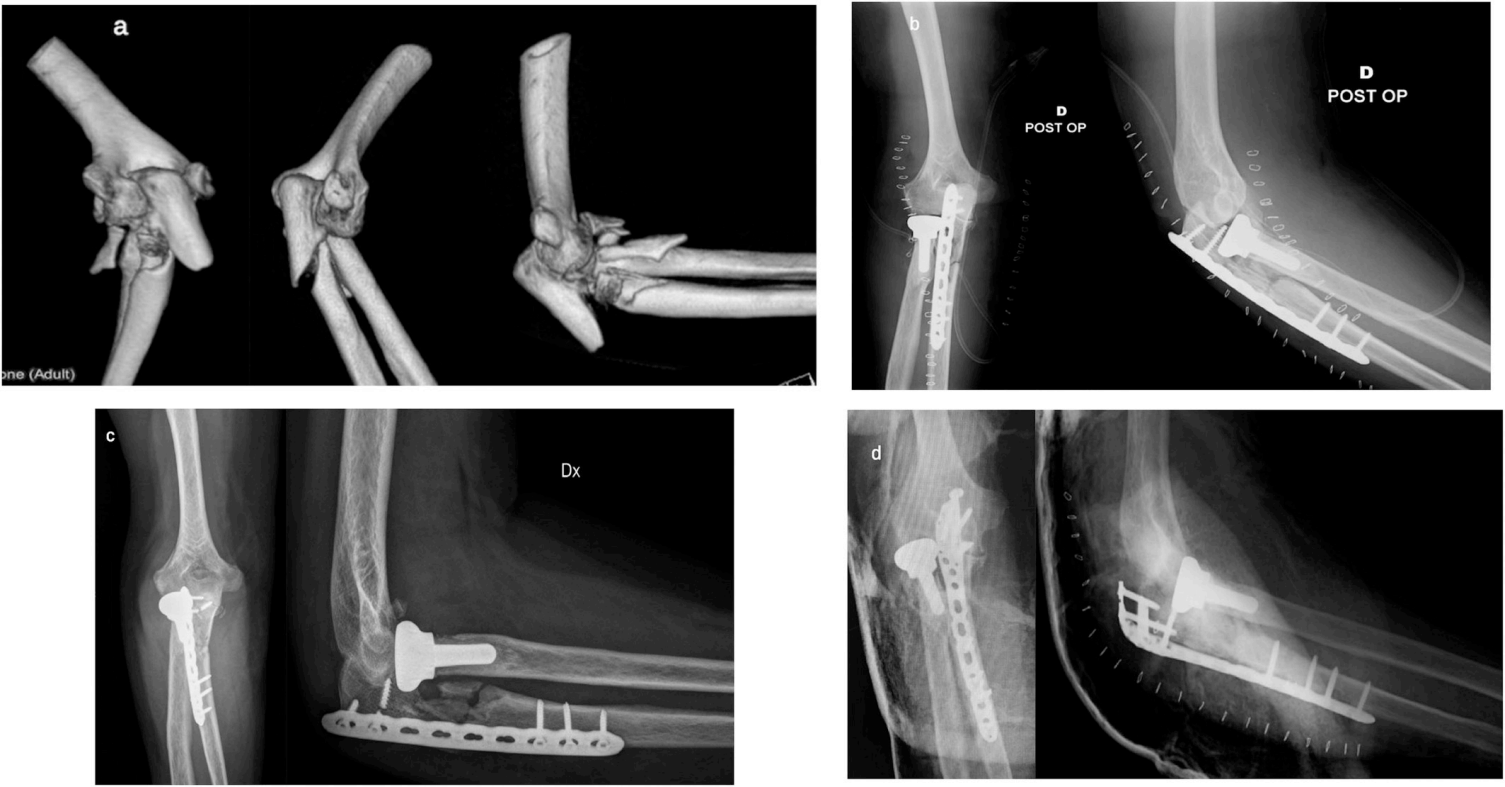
a) 3D-CT scan: comminution of the proximal ulna, coronoid and of the radial head in osteoporotic bone in a 72-years old woman. b) Post-operative radiographs: global posterior approach - initial attempt to perform anatomical synthesis of comminuted osteoporotic fragments with consequent biological damage – Weak final bridging plate fixation (No locked screws). - cemented radial head prosthesis. c) Radiographs at 3 months after surgery: Breakage of one screw, implant loosening. d) Post-operative radiographs after revision surgery: Pre-contoured bridging plate, iliac crest graft.
